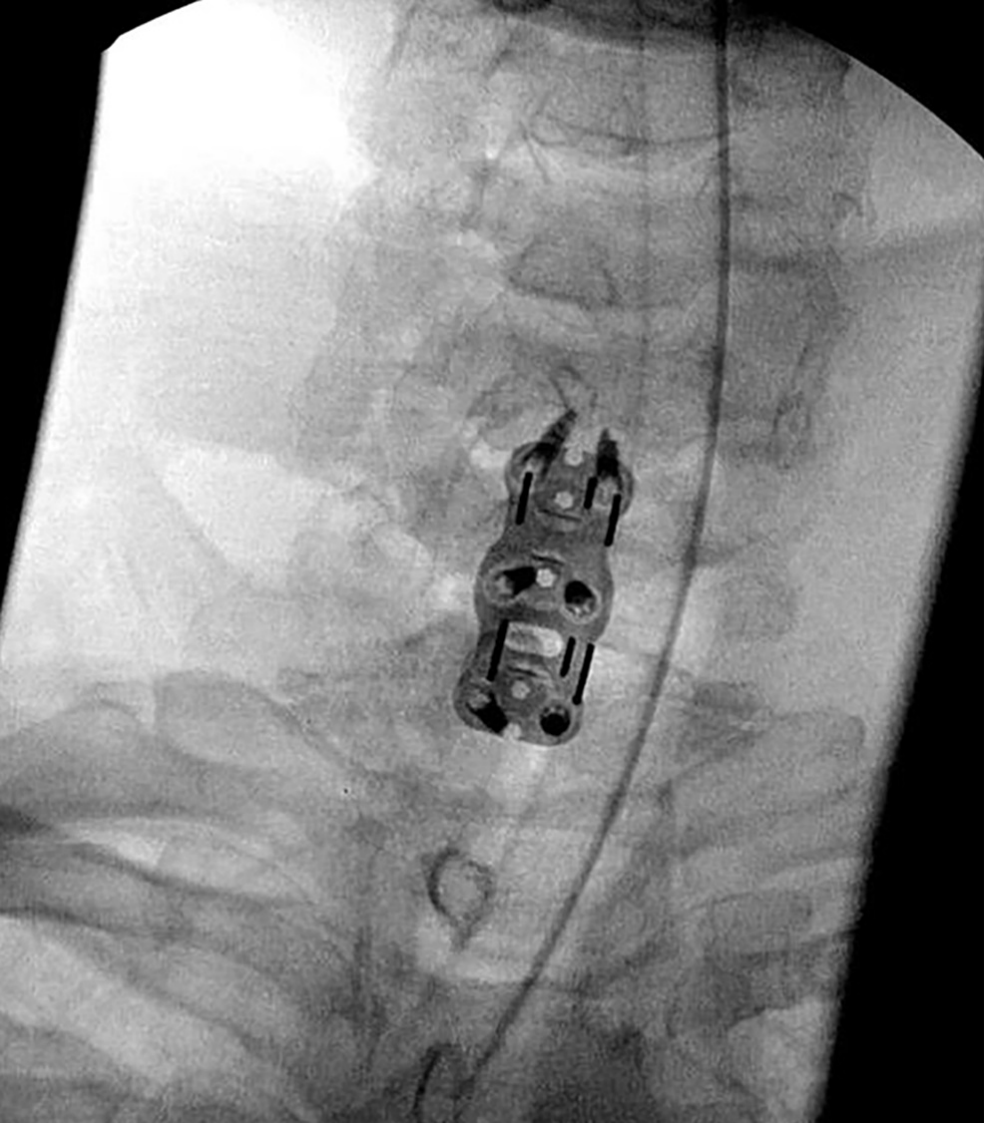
X-ray cervical spine post-ACDF plate placement ACDF: anterior cervical discectomy and fusion
